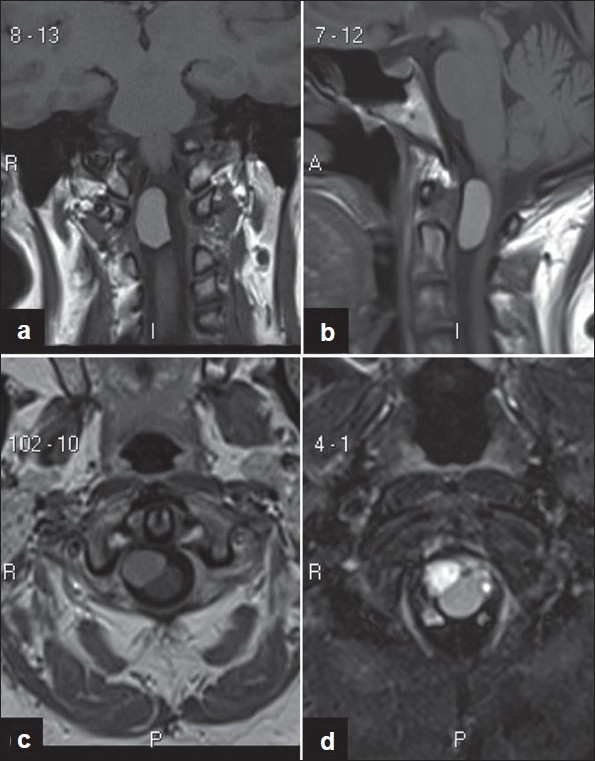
MRI of an intradural/extramedullary neurenteric cyst located at the craniocervical junction. Coronal (a) and sagittal (b) T1-weighted MRI showing the ventrally located homogenous lesion compressing the cervical spinal cord. Axial imaging demonstrates cord flattening by the anteriorly located neurenteric cyst hyperintense on both T1-weighted (c) and T2-weighted (d) MRI
